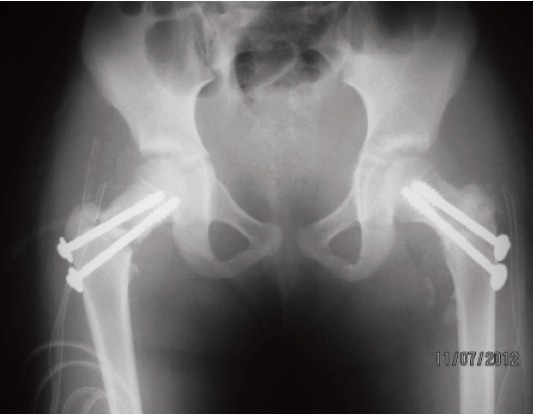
Anterioposterior radiograph of pelvis after internal
